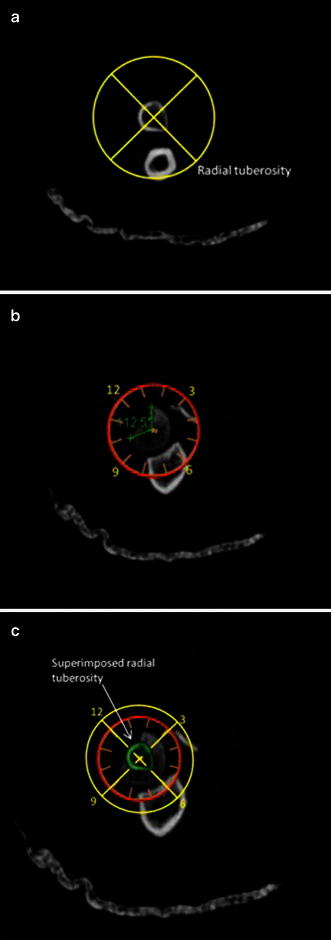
a Axial CT scan best representing the radial tuberosity. b Axial CT scan of radial head with superimposed clock face and measured angles of missing radial head fracture. c Axial CT scan of radial head and superimposed radial tuberosity
